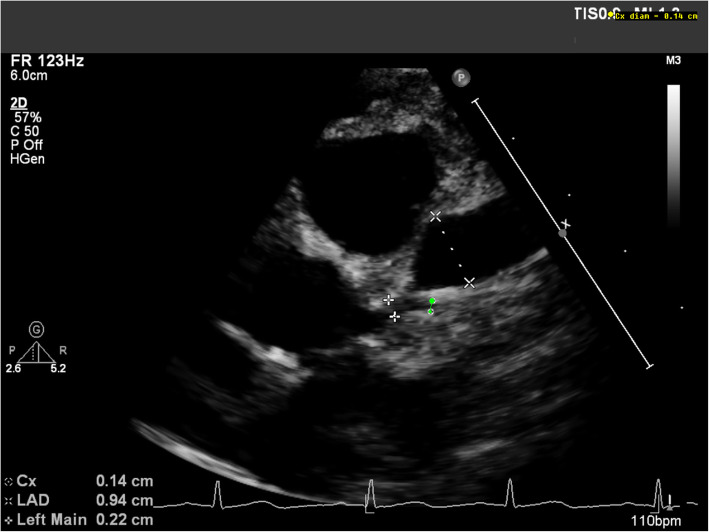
Coronary artery aneurysm in a MIS-C patient (Case 7). Echocardiographic short axis view of the left coronary artery system showing the mildly dilated main left coronary artery and the giant aneurysm in the left anterior descending coronary artery with the respective measurements (Video in the supplemental file). LMCA: Left Main Coronary Artery; Cx: circumflex; LAD: Left Anterior Descending
